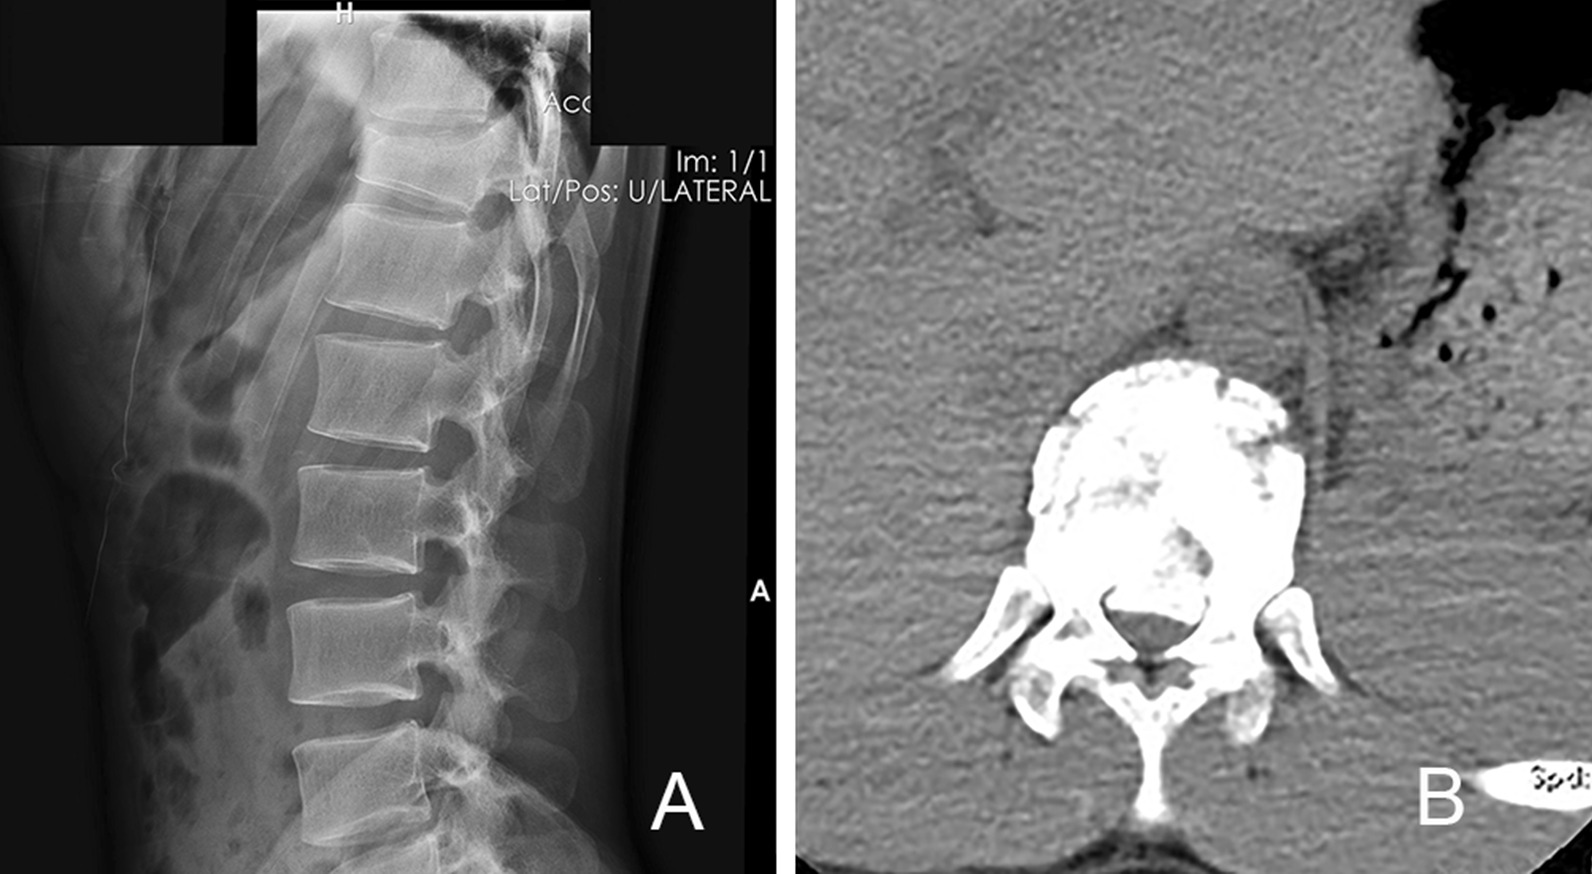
Radiograph of a 30-year-old patient who sustained a T11 burst fracture after a fall (A). Computed tomography showed that a large bone fragment had herniated into the spinal canal (B)
